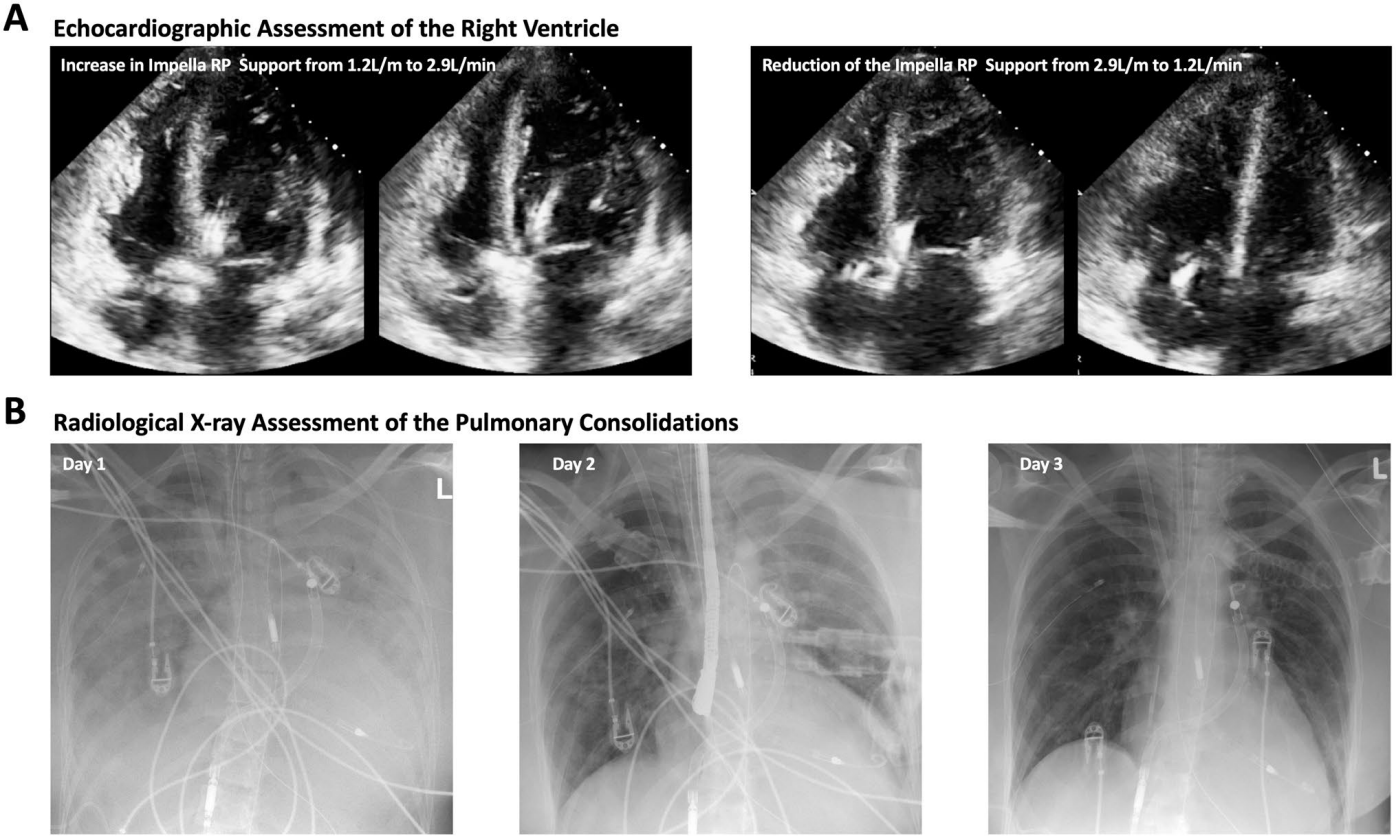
A Echocardiographic assessment showing immediate improvement as well as deterioration of the right ventricular function and dimensions depending on the Impella RP^®^ flow. B X-ray controls showing the initial pulmonary edema and consolidations on day 1, that significantly regressed over time using the Impella RP^®^ assist device
